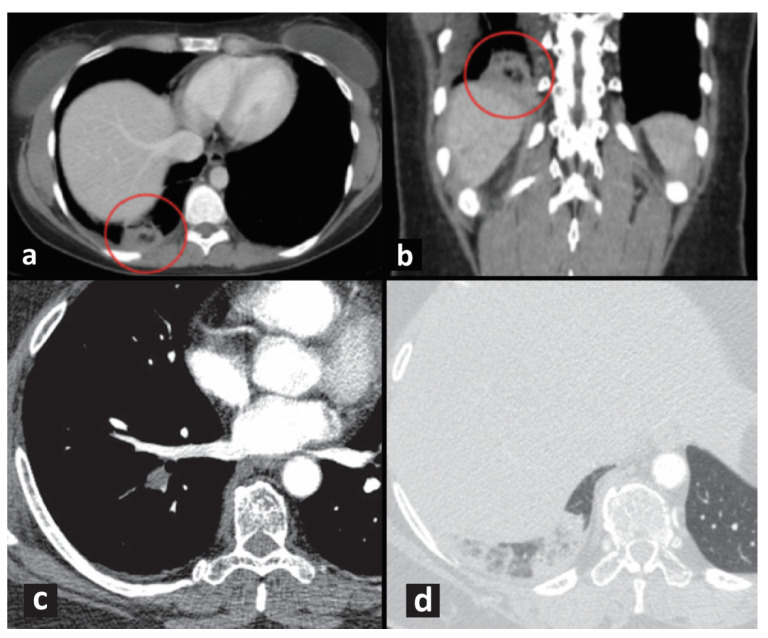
(a,b) CT of typical “bubbly consolidation” with central lucencies, indicated by red circles. (c) CT pulmonary angiography shows a filling defect within a segmental artery of the right lower lobe (acute pulmonary embolism). (d) Typical findings of a pulmonary infarction are better recognizable on images reconstructed with a parenchymal filter: a peripheral pleural-based consolidation with convex borders (Hampton hump) and small central clearance, without air bronchogram in the lower right lobe.
